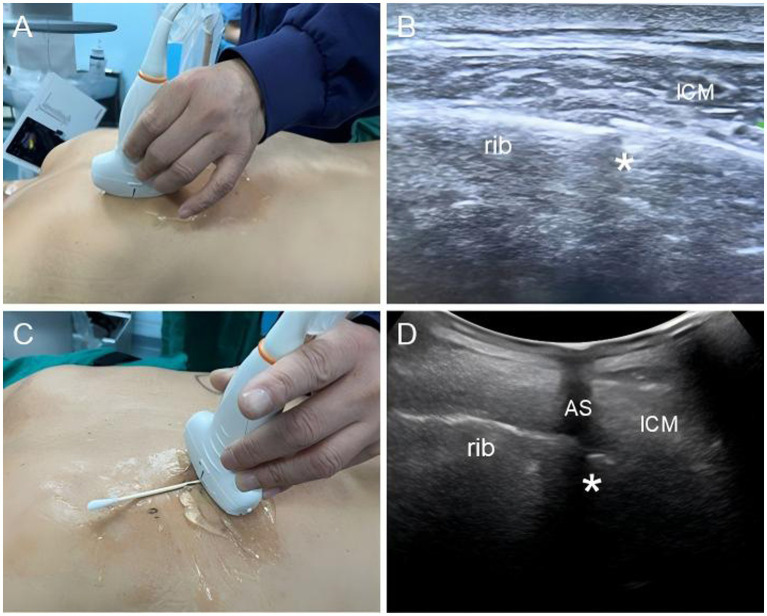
DASE technique for pre-incision rib localization. (A) Pre-incision ultrasound scanning along the rib surface. (B) Sonographic identification of a rib fracture, shown as cortical disruption of the rib surface with an associated acoustic shadow; the asterisk indicates the fracture site. (C) Placement of a cotton swab on the skin beneath the probe to generate an external acoustic reference shadow. (D) Alignment of the externally generated acoustic shadow with the identified fracture site to translate the subsurface finding into a skin-surface mark for incision planning. ICM, intercostal muscle; AS, acoustic shadow.
